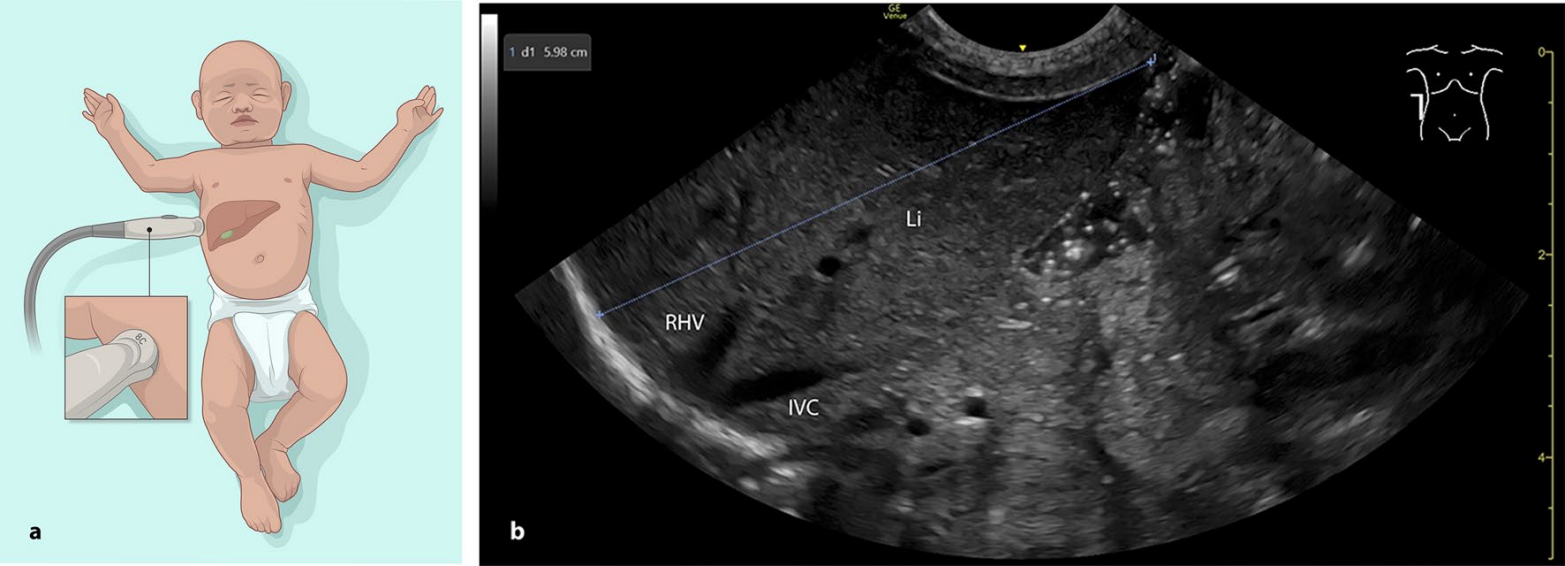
Measuring the liver length in the right AAL in the lateral plane. a infant in supine position with a convex probe positioned in the right AAL from the flank (lateral-medial plane). b sonographic craniocaudal liver length measurement. AAL: anterior axillary line, IVC: inferior vena cava, Li: liver, RHV: right hepatic vein
